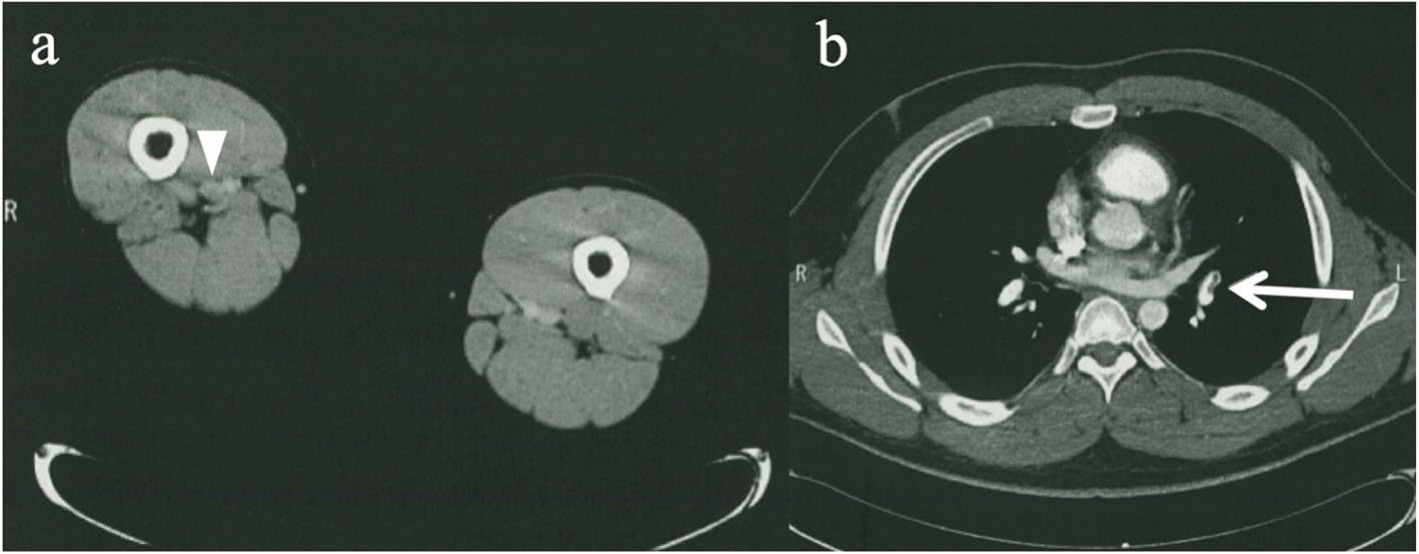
Contrast-enhanced CT was read by radiology specialists to obtain a definitive diagnosis of DVT (a; arrowhead) and PE (b; arrow)
